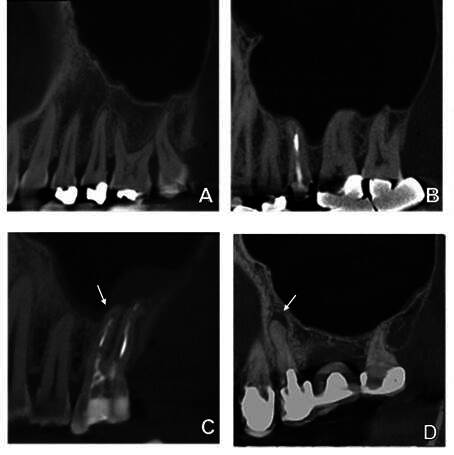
Periradicular conditions. (A) All teeth have an intact lamina dura. (B) The lamina dura of all teeth is fused with the maxillary sinus floor in their periapical region. (C) Discontinuation of the lamina dura on the mesiobuccal root of tooth 26. (D) Apical periodontitis on tooth 25.
